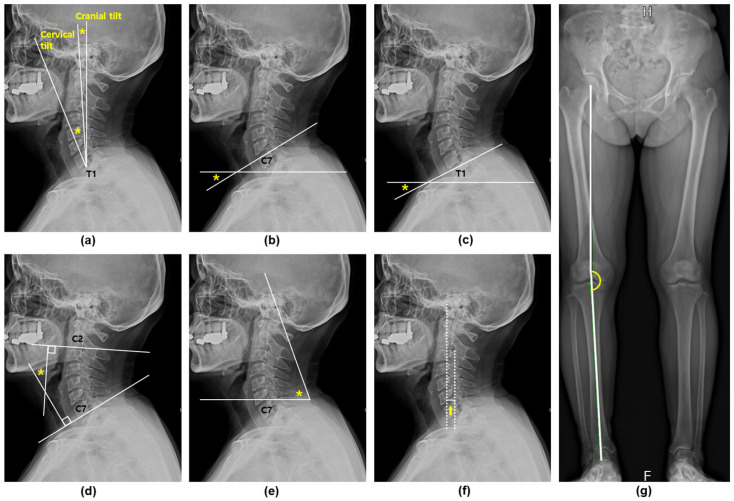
Radiographic assessment of cervical spine and lower-limb alignment using cervical lateral X-ray and EOS anteroposterior radiographs. (a) Cervical tilt and cranial tilt. (b) C7 slope. (c) T1 slope. (d) C2–7 Cobb angle. (e) Craniovertebral angle. (f) C2–7 sagittal vertical axis. (g) Radiographic hip–knee–ankle angle. * Indicates the locations where the angles shown in (a–e) were measured on the radiographs. The arrow indicates the C2–7 sagittal vertical axis in panel (f).
